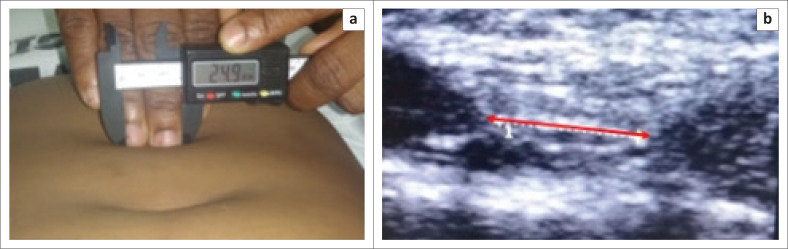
Measurement of interrecti distance: (a) caliper measurement and (b) ultrasound measurement.
